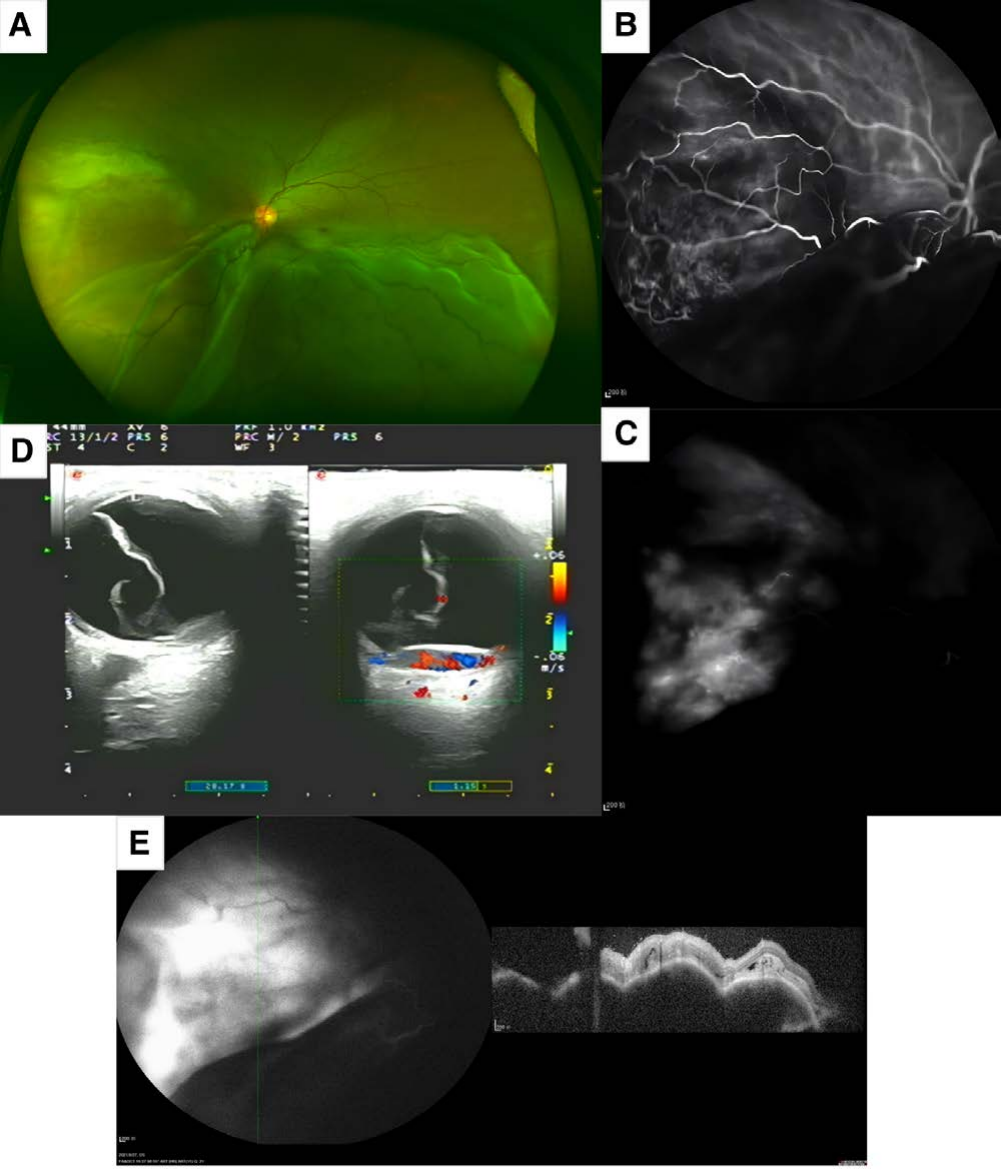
Fundoscopy, ICGA, Doppler ultrasound and OCT examinations of the patient at presentation. Ultra-wide-field fundus photography showed inferior retinal elevation and a dome-shaped, reddish-white choroidal mass nasal to the optic disc (A). ICGA showed early-stage mottled hyper- and hypofluorescence in the nasal region (B), with late-stage diffuse hyperfluorescence interspersed with areas of hypofluorescence, accompanied by leakage (C). Doppler ultrasound identified a nasal choroidal mass with a basal diameter of 14.0 mm and a thickness of 0.42 mm, exhibiting abundant blood flow signals (D). OCT revealed choroidal elevation, significant compression of the choriocapillaris, and disruption of the photoreceptor layer (E). ICGA = indocyanine green angiography, OCT = optical coherence tomography.
